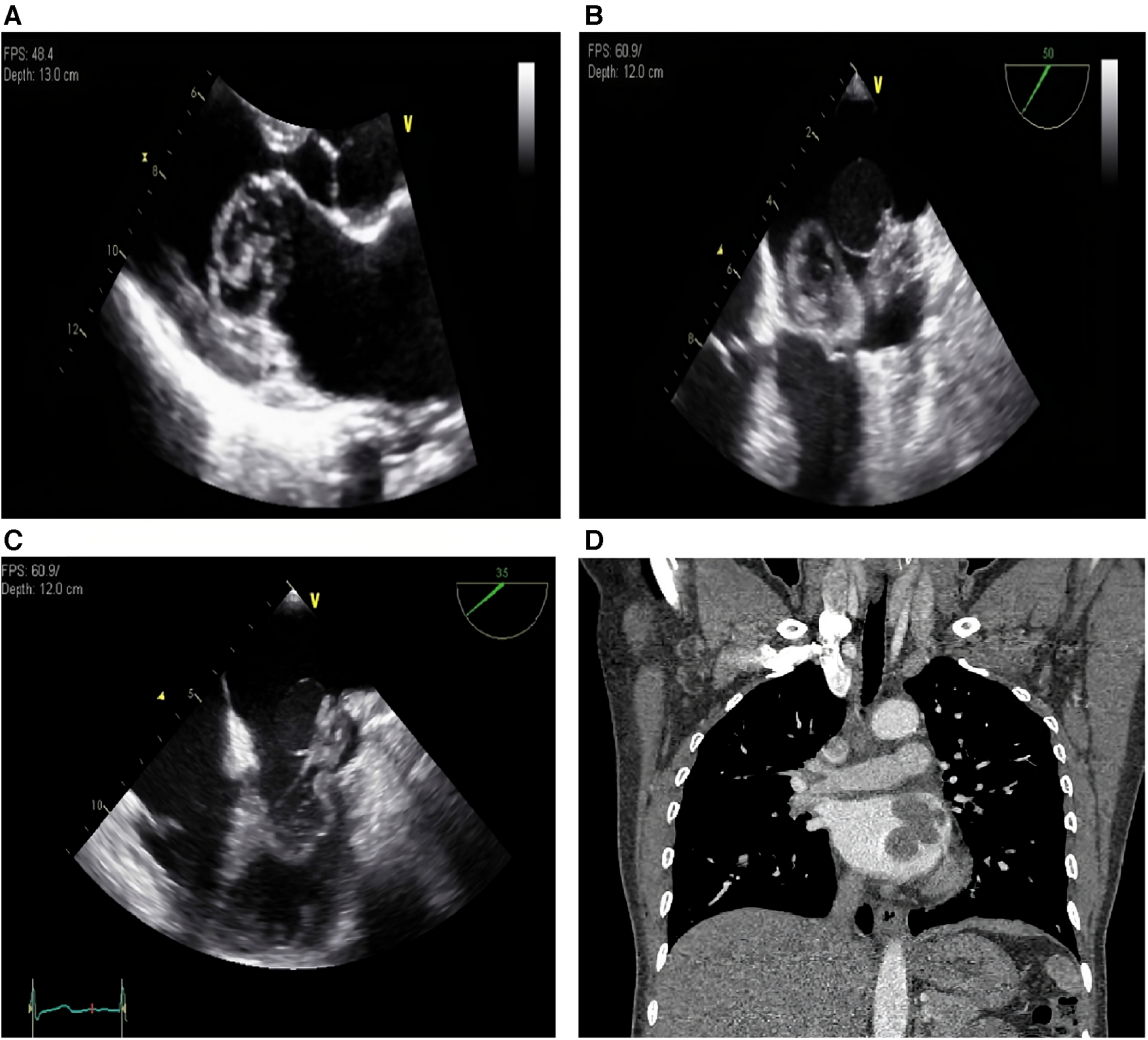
(A) Transthoracic echocardiography, parasternal long axis view. A broad-based mass is filling the left atrium and protruding into the LV cavity during diastole. (B) Transesophageal echocardiography, systole. The mass which is composed of three different parts are shown. (C) Transesophageal echocardiography, diastole. The mixed portion of the mass is protruding into the LV cavity during diastole. (D) Thoracoabdominal computed tomography reveals an intracardiac mass occupying the left atrium. LV, left ventricle.
